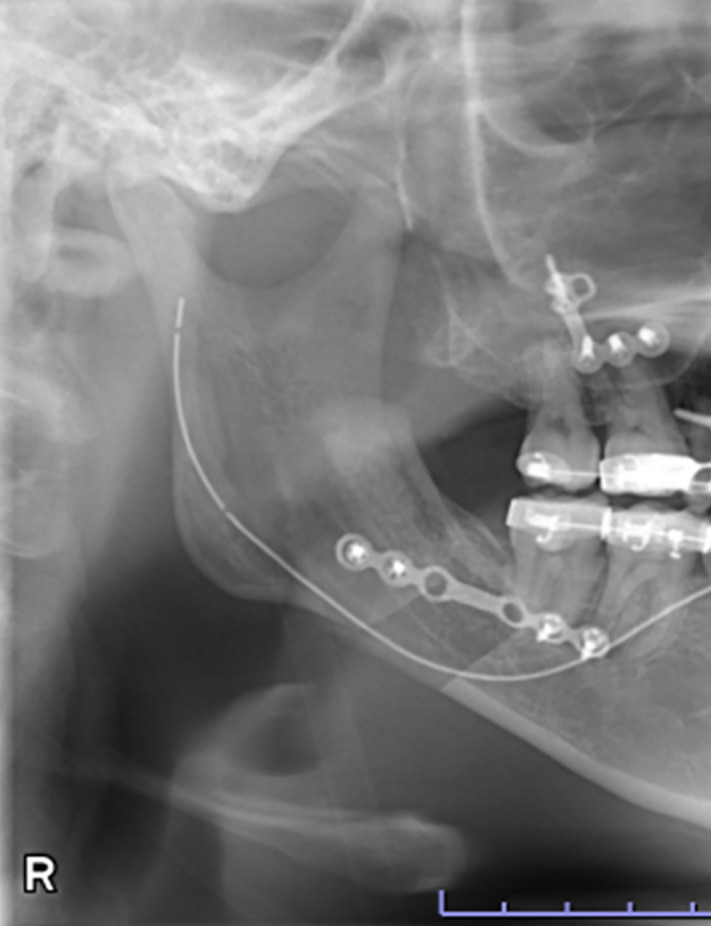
Panoramic radiograph showing the lateral osteotomy line in Obwegeser–Dal Pont method.
